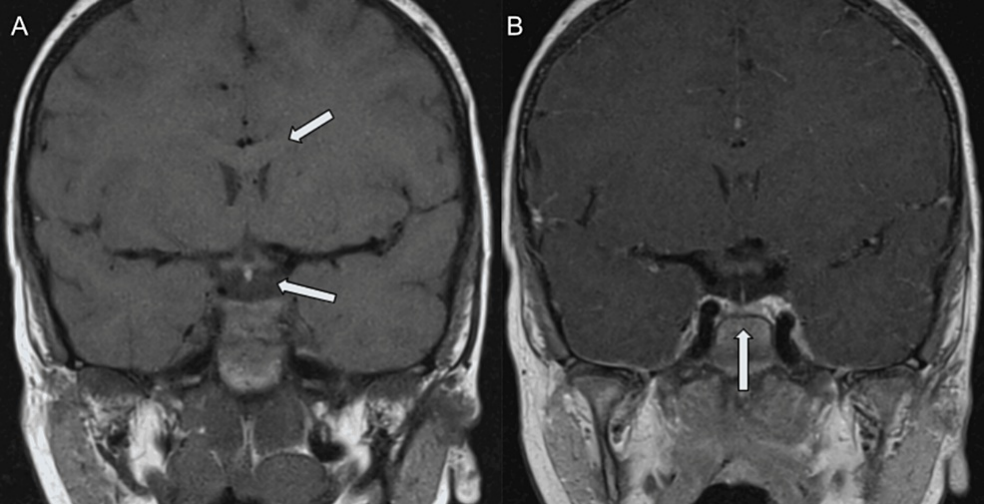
Infundibulum and pituitary hypoplasia on coronal T1 MRI A) Anterior to the callosal body agenesis, T1-weighted image (T1WI) appreciates the presence of commissural genu fibers, and B) a thin infundibulum and hypoplastic pituitary gland.
